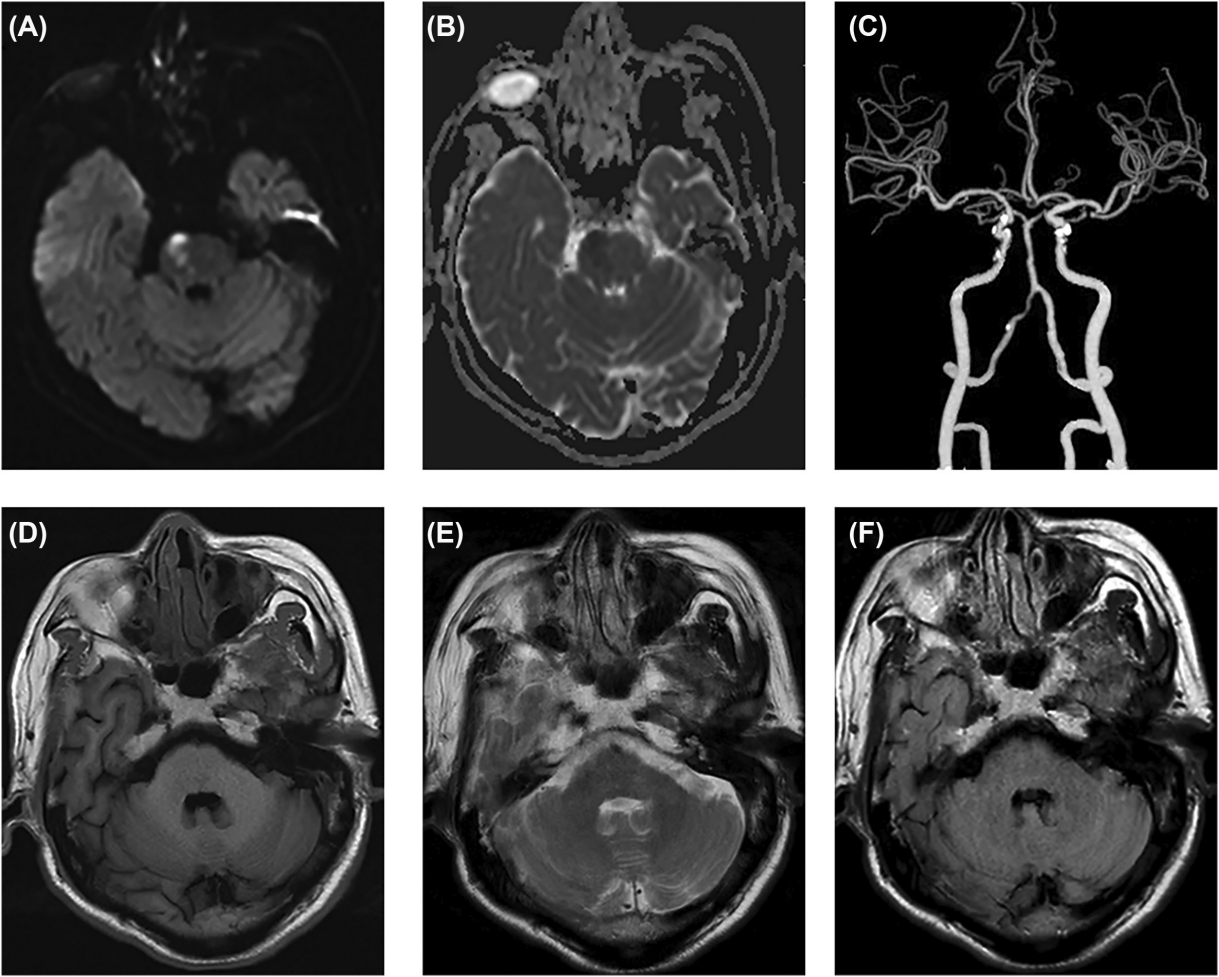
The initial MRI examination of patient 1 revealed the presence of an acute infarction in the right pons. (A, B) exhibited high signal intensity on diffusion‐weighted imaging (DWI), and low signal intensity on the apparent diffusion coefficient (ADC) map, in the right anterior pons. Computed tomography angiography (CTA) demonstrated the presence of multifocal stenosis in both the right vertebral artery (V4 segment) and basilar artery (C). (D) Axial T1 weighted image, (E) axial T2 weighted image and (F) axial FLAIR weighted image did not exhibit any abnormal signal patterns in the bilateral middle cerebellar peduncles.
